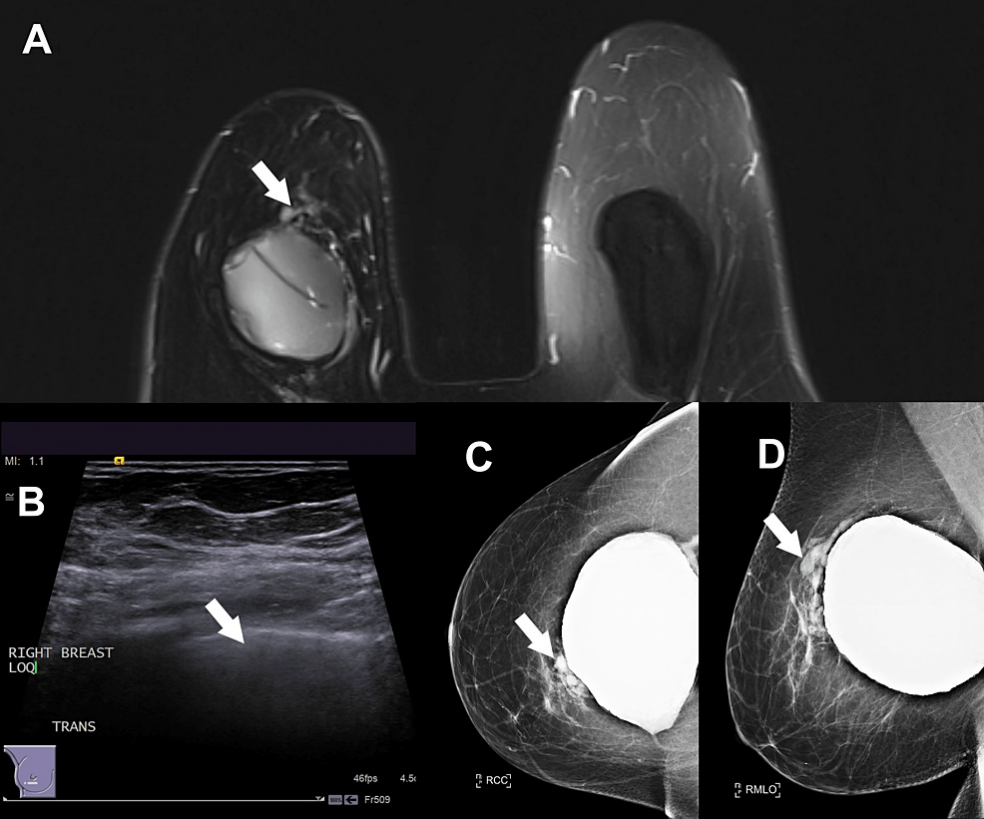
(A) Magnetic resonance imaging shows intracapsular and extracapsular rupture. (B) Ultrasound of the affected area revealed dispersion consistent with the presence of extracapsular silicone. (C) Craniocaudal and (D) mediolateral oblique mammogram images are consistent with implant rupture and the presence of extracapsular silicone.
